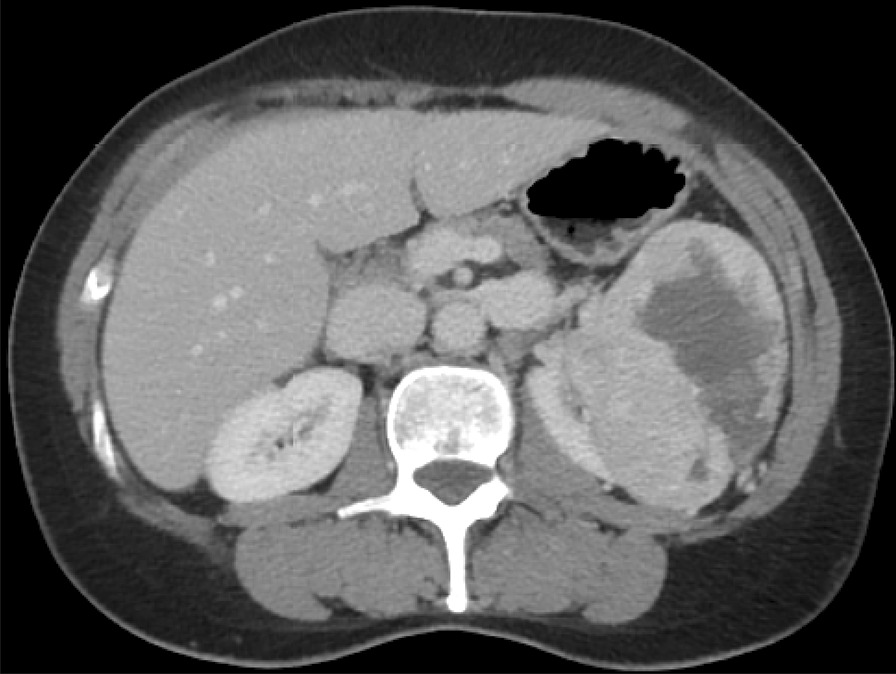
Computed tomography image of the left renal mass at diagnosis.
